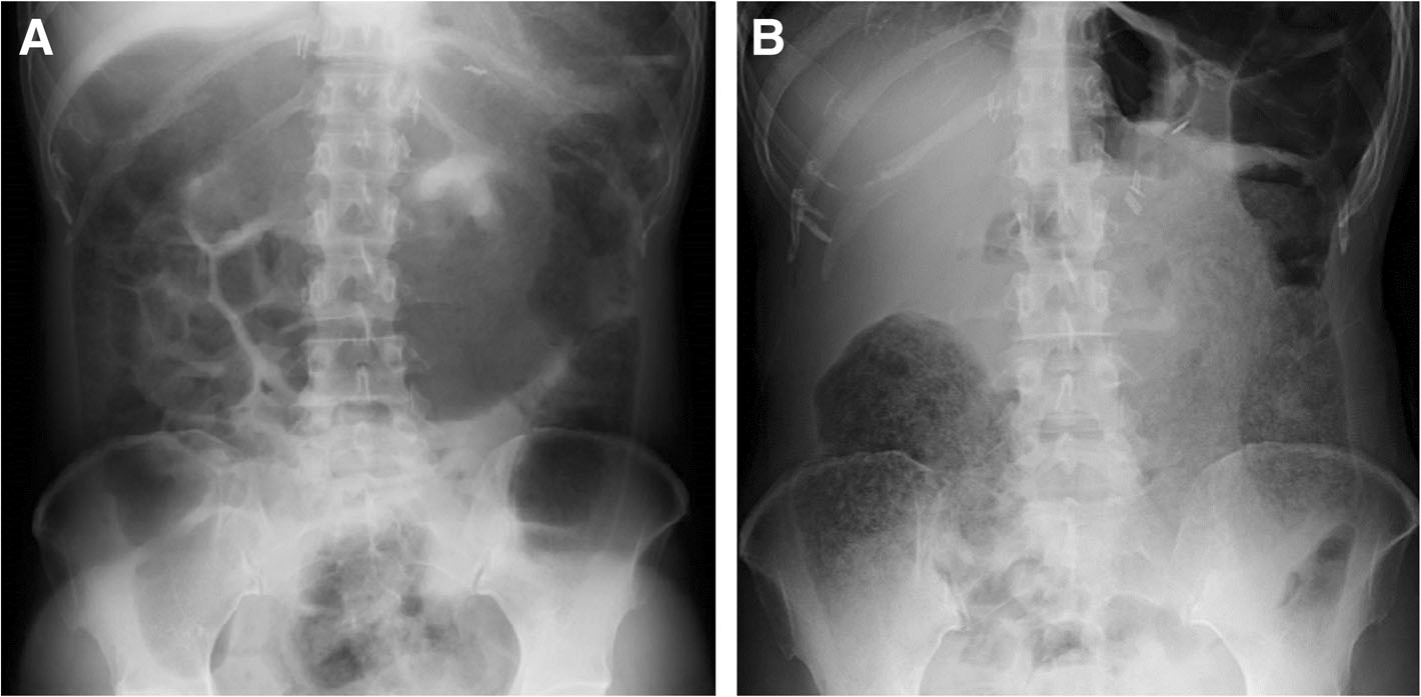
Abdominal radiograph on admission (a) and on postoperative day 30 (b). Remarkable distended bowel and gas on admission was relieved on postoperative day 30
